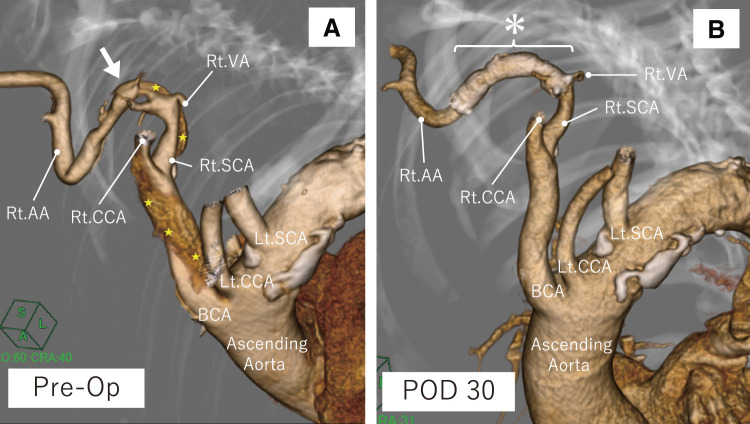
Fig. 1 Three-dimensional reconstructions of computed tomographic angiography before and after the procedure. (A) A markedly tortuous Rt. SCA is observed, with the entry tear identified distal to the Rt. SCA. (B) The Rt. VA remains patent, and the stent graft is positioned appropriately. Arrow: entry; Star: false lumen; Asterisk: stent graft. Rt.: right; Lt.: left; BCA: brachiocephalic artery; CCA: common carotid artery; SCA: subclavian artery; AA: axillary artery; VA: vertebral artery; Op: operation; POD: postoperative day
